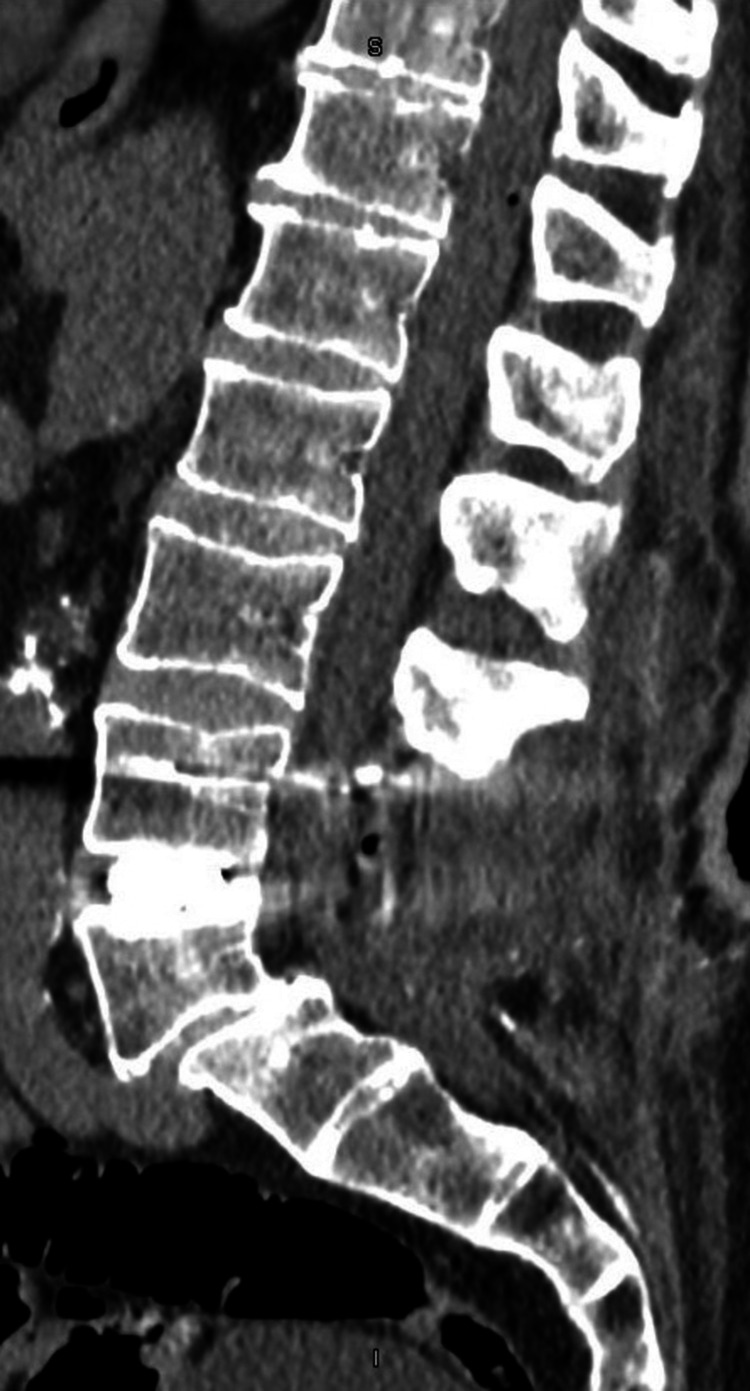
Postoperative sagittal CT scan shows new, larger laminectomies at L4 and L5 with proper placement of new disc prosthesis and new pedicle screws at L4 connected to previously placed pedicle screws at L5.
